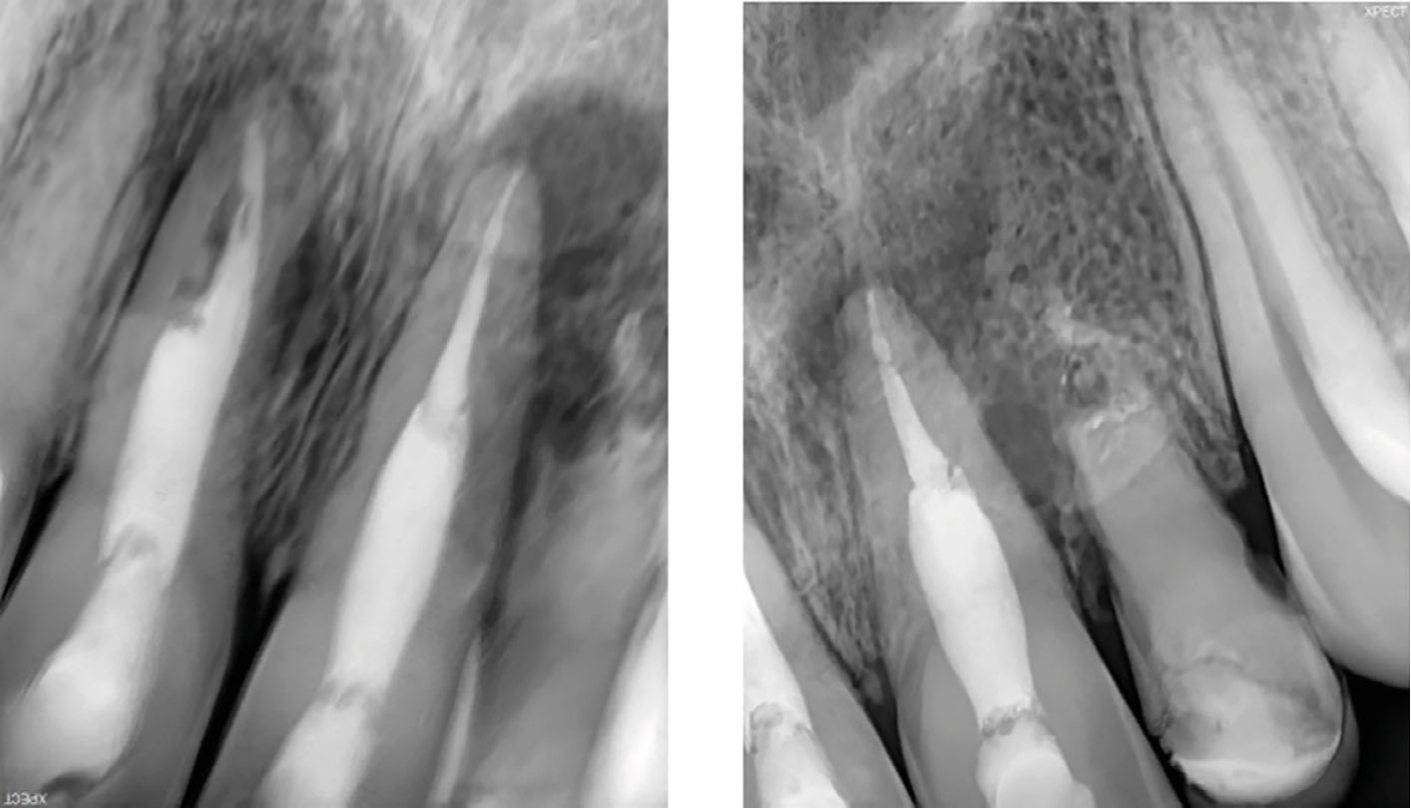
Radiographic images of obturation done in relation to Tooth Numbers 11 and 21 using MTA and in Tooth Number 22 using Biodentine.
